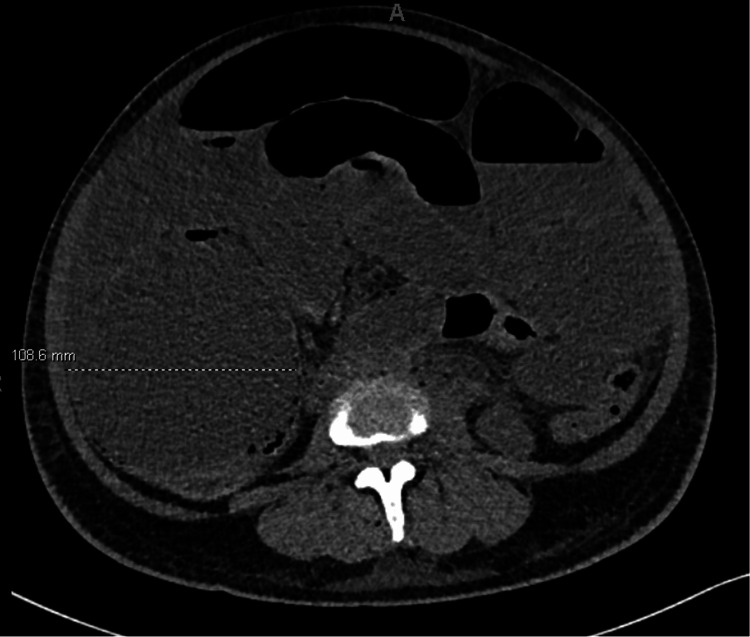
Coronal views of CT abdomen/pelvis showing a dilated cecum to 10.9 cm diameter
